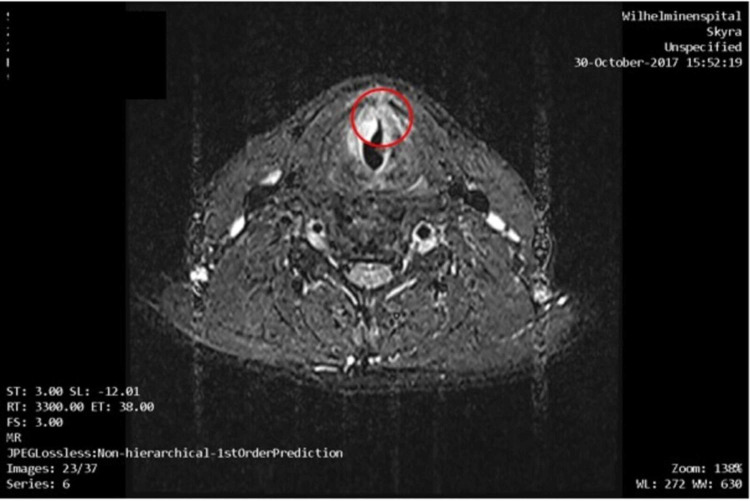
MRI with contrast agent from October 2017. Soft tissue formation (13 mm x 9 mm x 13 mm) at the commissura anterior of the vocal cord on the left with contrast enhancement and diffusion restriction, showing the recurrent tumor, approximately the same size as in previously performed CT. Additionally, there is a bilateral swelling of the vocal cords, caused by the tumor with progressive narrowing of the airways in comparison to the previously performed CT. Lymph nodes increased in number and borderline in size in Level 1b, 2, and 3 on both sides.
